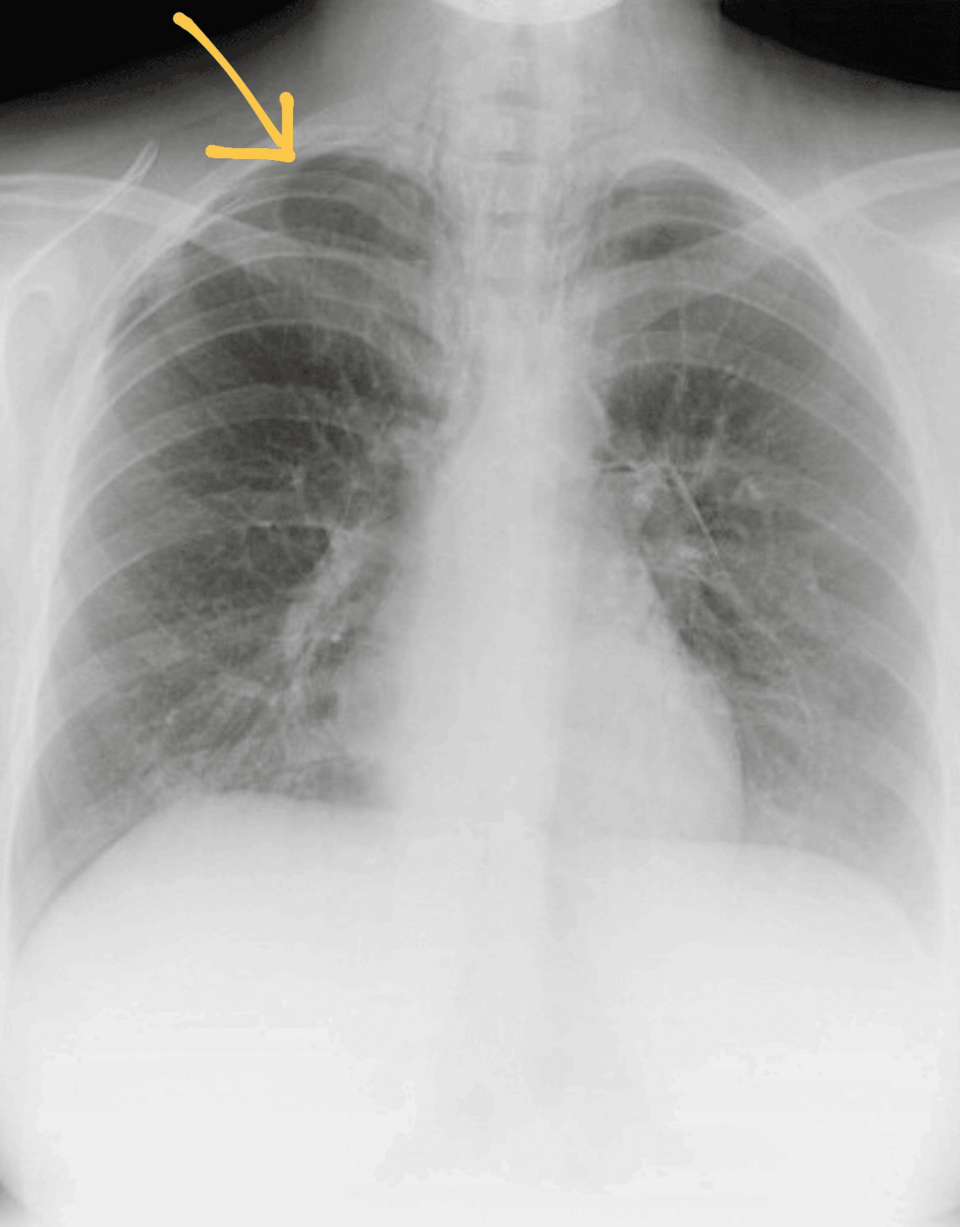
Chest radiograph showing right-sided pneumothorax with mediastinal air.
